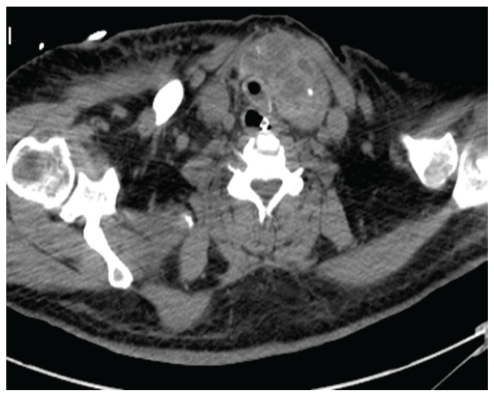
A CT in a patient with a short neck and with a very large goiter. The CT cut is approximately 1 cm above the sternal notch. The trachea is surrounded by the goiter and cannot be safely reached from the anterior portion of the neck, unless thyroidectomy is performed in advance.
